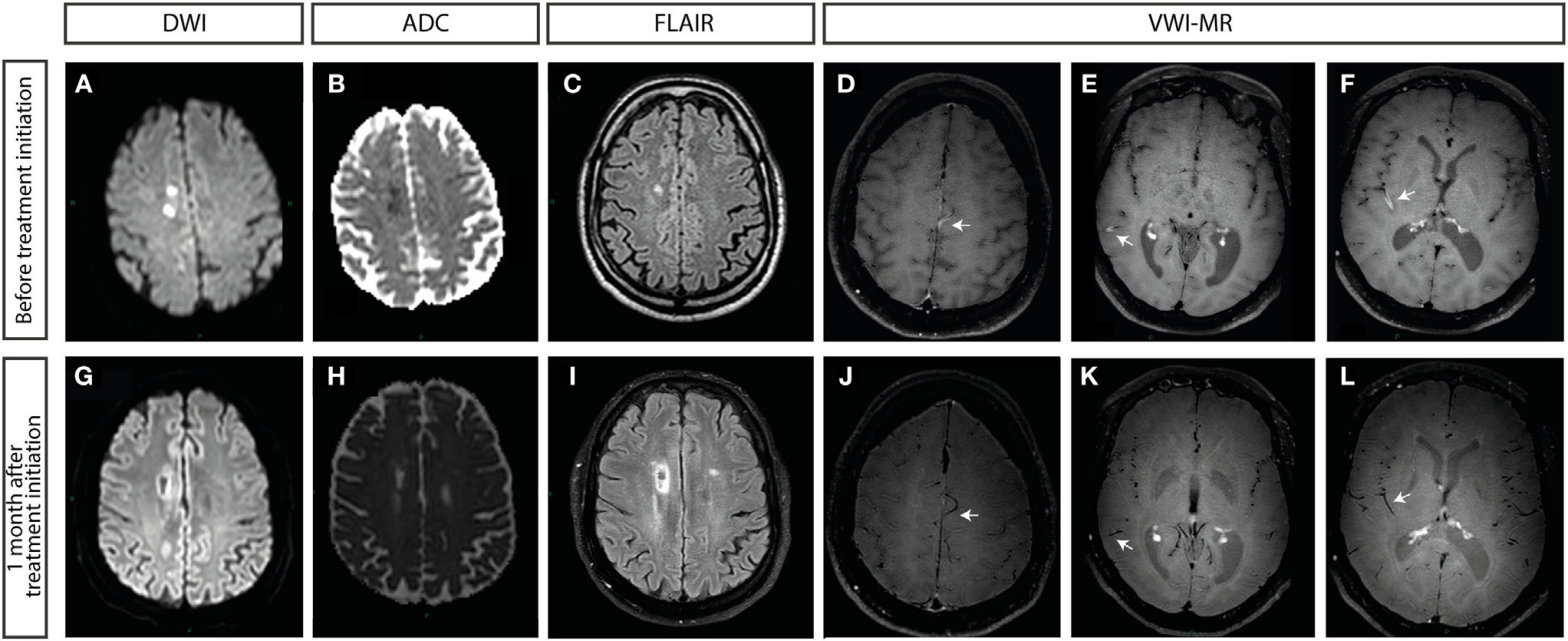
Brain MRI at admission (A–F) and 1 month after treatment initiation (G–L). (A,G) Diffusion-weighted imaging and (B,H) apparent diffusion coefficient showing restricted diffusion in the right anterior cerebral artery territory, compatible with a recent ischemic stroke. (C) FLAIR sequence showing postischemic lesions in the anterior territory. (I) Extension of the lesion in FLAIR confirming established lesions in right and left anterior cerebral arteries. (D–F,J–L) Vessel wall imaging with MRI (VWI-MR), black blood MRI [high-resolution (3-T), black-blood fat suppressed pre- and post-contrast T1 weighted images]. (D–F) Concentric contrast enhancements (CEs) suggesting a central nervous system vasculitis (indicated by arrows). (D) CE of a distal branch of the left anterior cerebral artery. (E,F) CE of distal branches of the right middle cerebral artery. (J–L) Retraction of the CE after 1 month of combined antiretroviral treatment.
